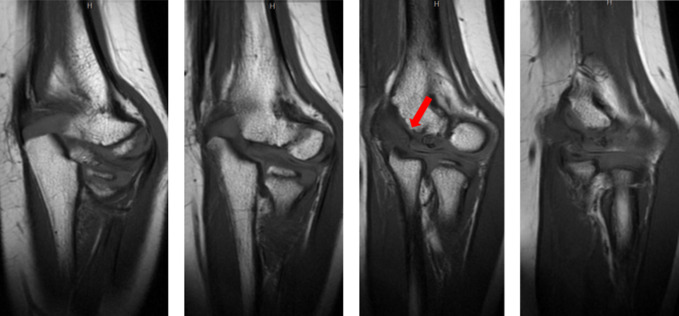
Radiographs demonstrating the sequential coronal T1-weighted MRI images of the left elbow. Breakdown of normal bony surface of the medical distal humerus shown (red arrow).
